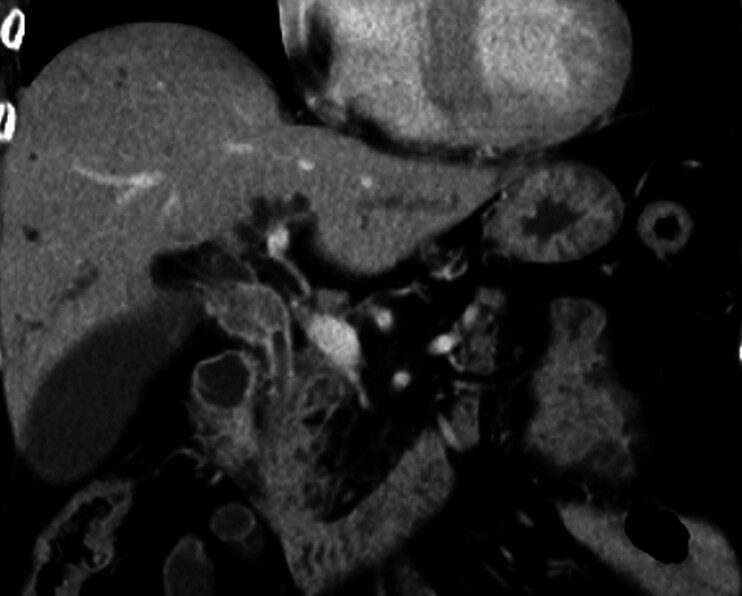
Computed tomography at the time of diagnosis. A mass was identified in the common bile duct, with dilation of the bile duct upstream from the mass.
